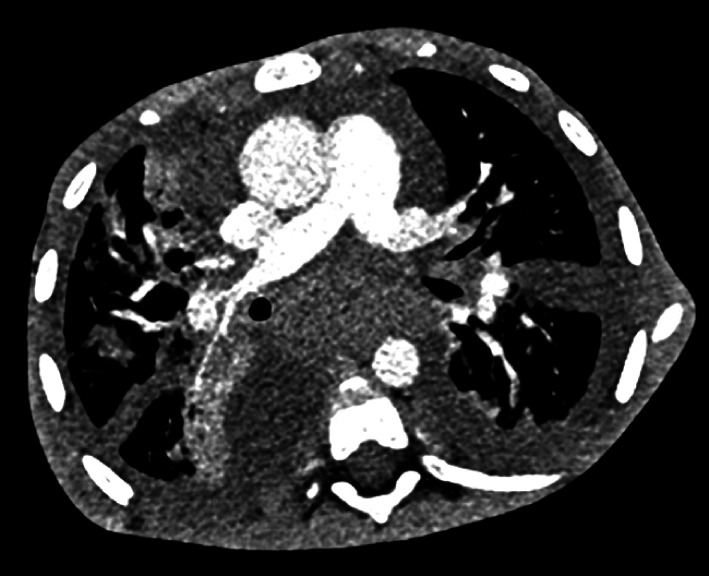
Computed tomography showing mediastinal lymphadenopathy and pulmonary changes in a 6-year-old liver transplant recipient.
